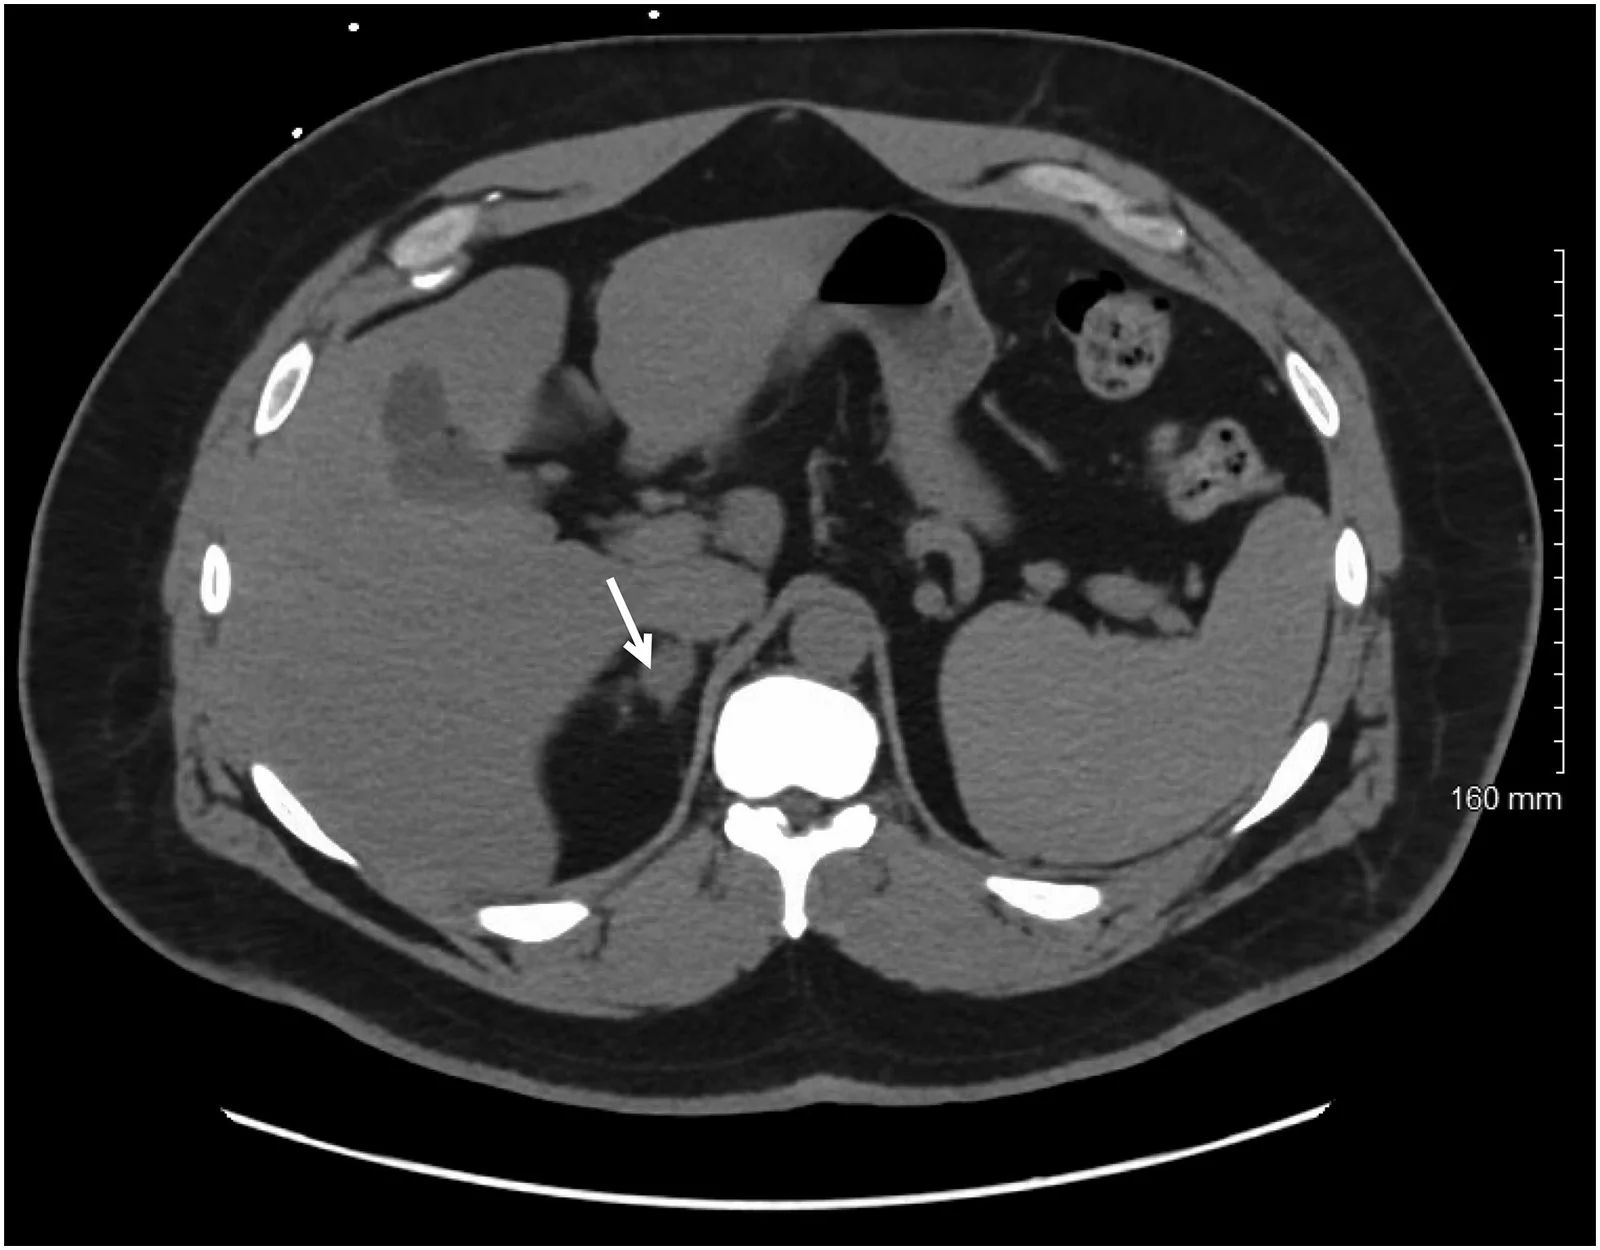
Computed tomography abdomen pelvis without contrast: stable right adrenal nodule with mild periadrenal stranding, obtained 3 months following adrenal vein sampling procedure.
